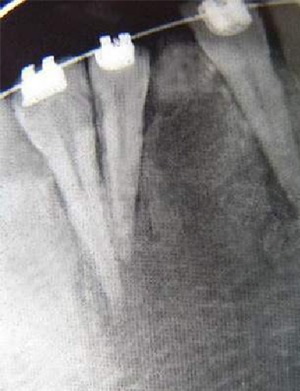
The periapical radiograph of our case showing widening of the periodontal ligament space and a coarse trabecular pattern. These important features were neglected.
